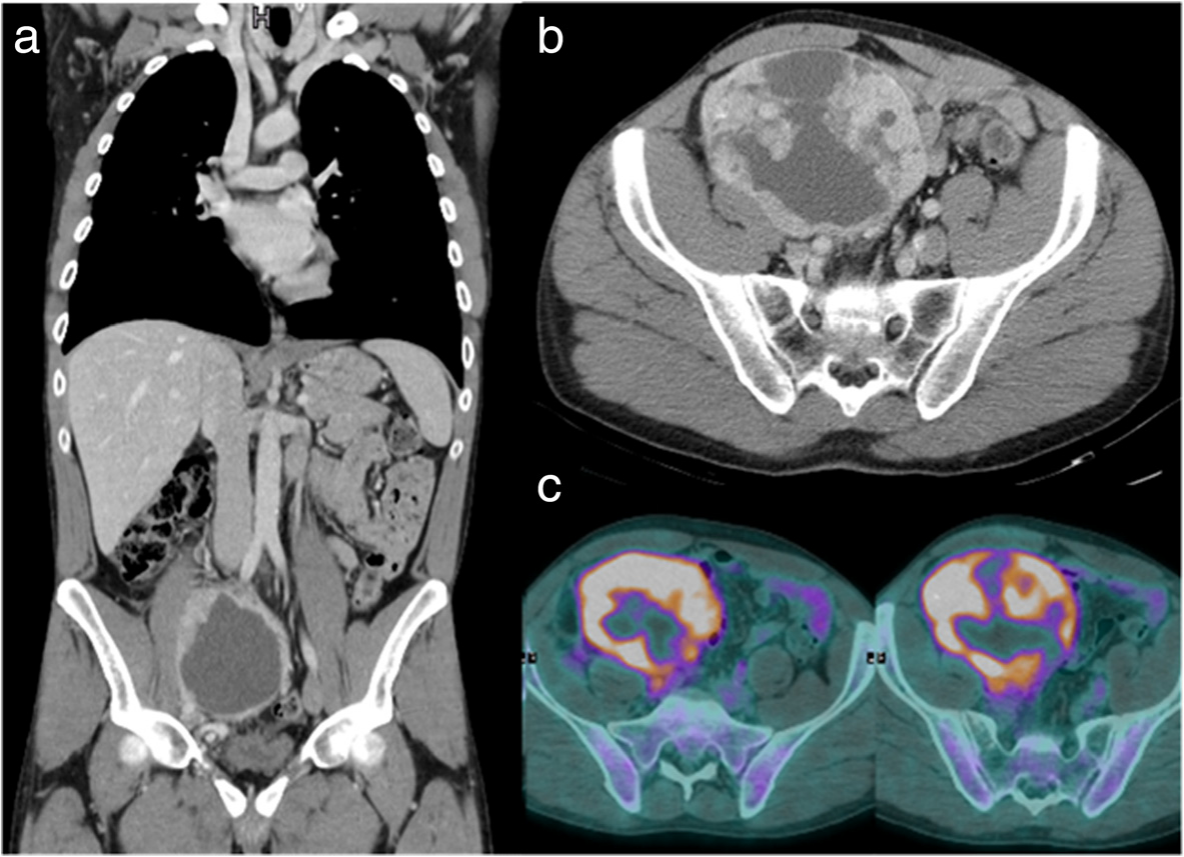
Coronal CT imaging. Coronal CT imaging showed a large mass occupying most of the lower portion of the right abdomen with a dyshomogeneous density due to cystic and necrotic areas and no infiltration of the surrounding tissues (a). The tumor is located near the omolateral outer iliac vein as well with secondary dislocation and suspect infiltration (b). In the PET/CT imaging, the central photopenic portion of the mass might reflect necrotic process (c).
